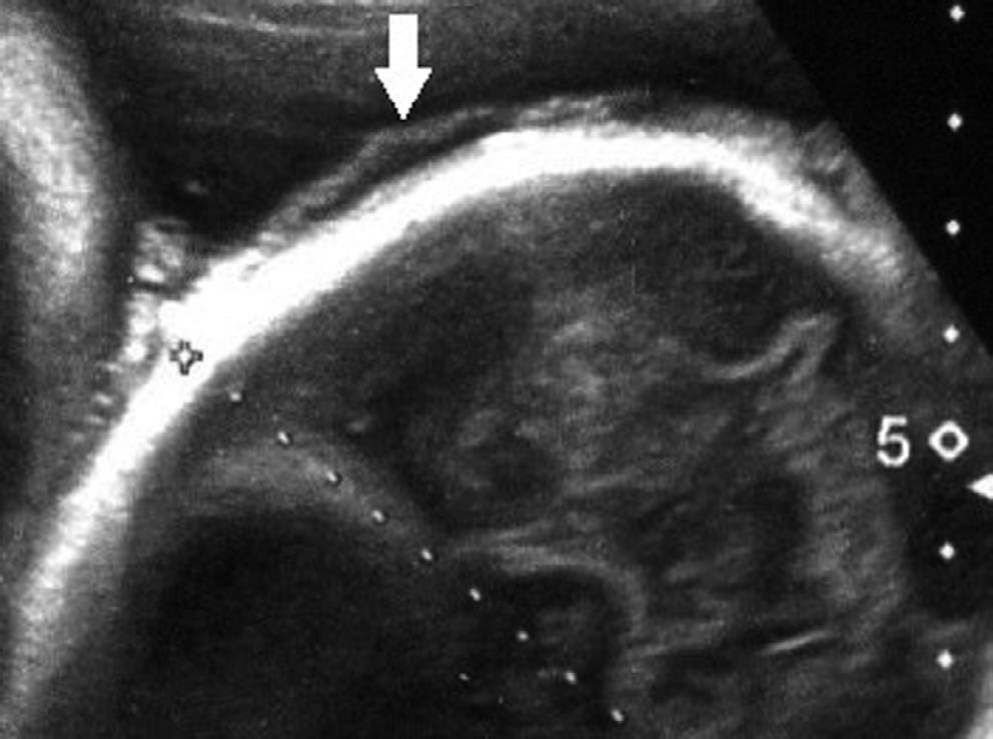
A fluffy, film‐like image detected near the head of the second twin by ultrasonography at 33 wk of gestation (white arrow)
